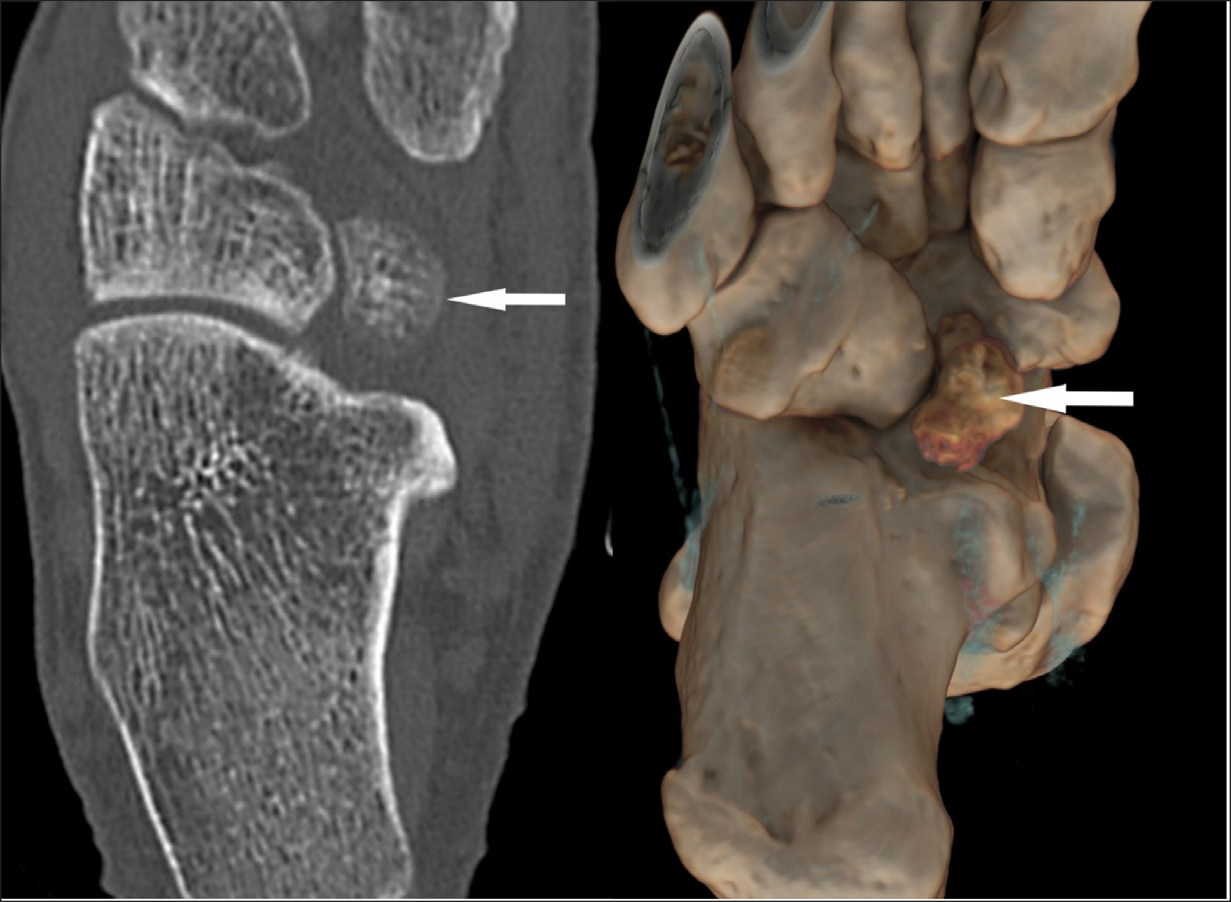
Despite the extensive bone marrow edema seen in the os cuboideum secundarium on MRI there was no fracture evident on CT. As there were no other abnormalities seen on MRI and CT imaging, bone marrow edema in the accessory ossicle and the plantar aspect of the talonavicular articulation are the most likely cause of the ankle pain. A stress reaction of the ossicle with the talonavicular articulation after trauma was the favored diagnosis.
